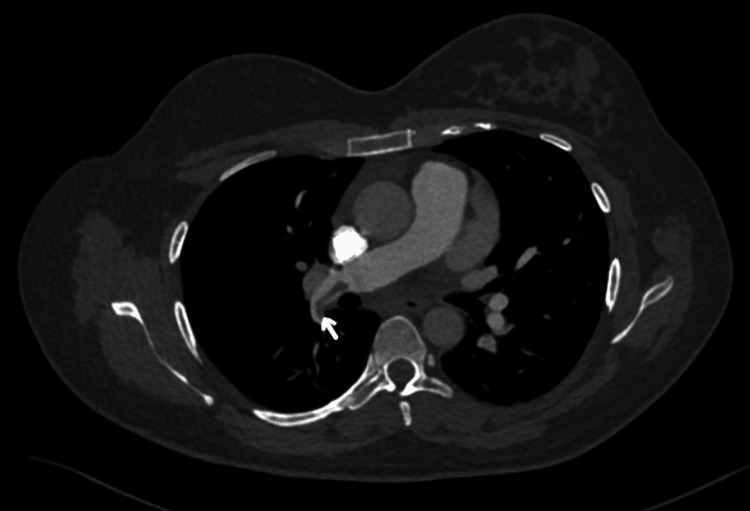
CT pulmonary angiogram (segmental branches of the right lung) There is evidence of a filling defect seen in the segmental branches of the right lung
